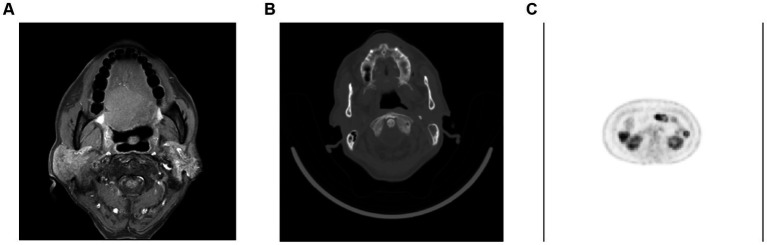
Samples of patients with HPV. (A) Head MRI of patients with oral squamous cell carcinoma, (B) CT of patients with oral squamous cell carcinoma, (C) PET of patients with cervical cancer.
